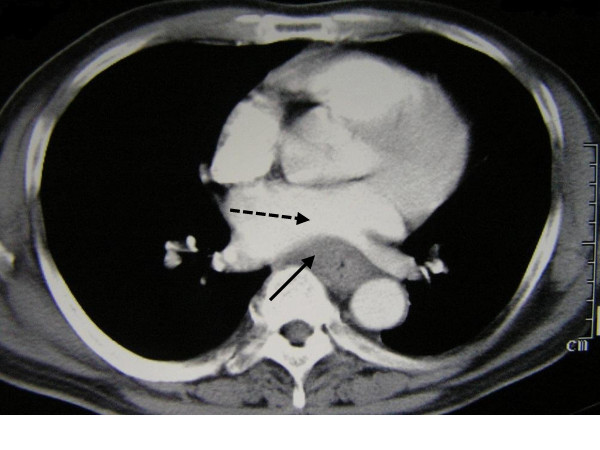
Computed tomography of the chest demonstrating esophageal thickening (compound line) compressing the left atrium (dashed line).
